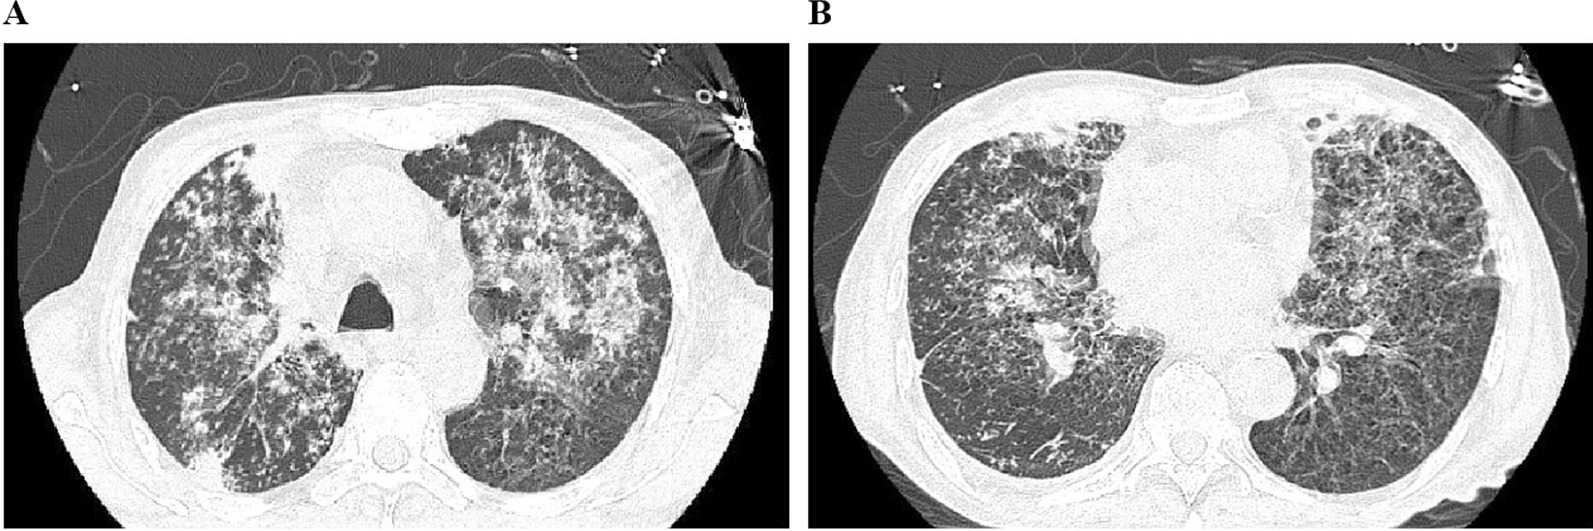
Images of chest CT on admission. A, B Chest CT scan showed multiple nodules and patchy infiltration in both lungs
